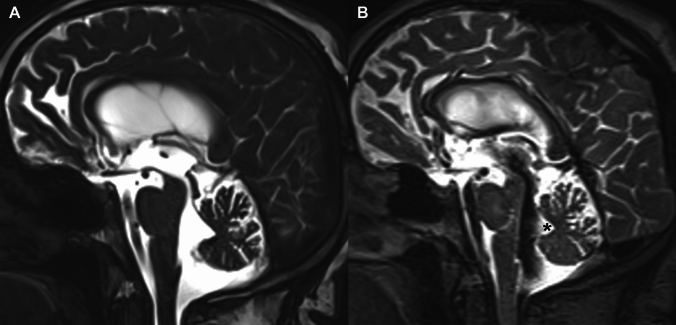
AB Sagittal T2-weighted CISS (A) and SPACE images (B) in a patient without iNPH. Lateral ventricles were also dilated in this patient. A thin line of signal void is seen within the fourth ventricle associated with normal CSF flow. Note the absence of flow at the level of the median dorsal recess (black asterisk)
